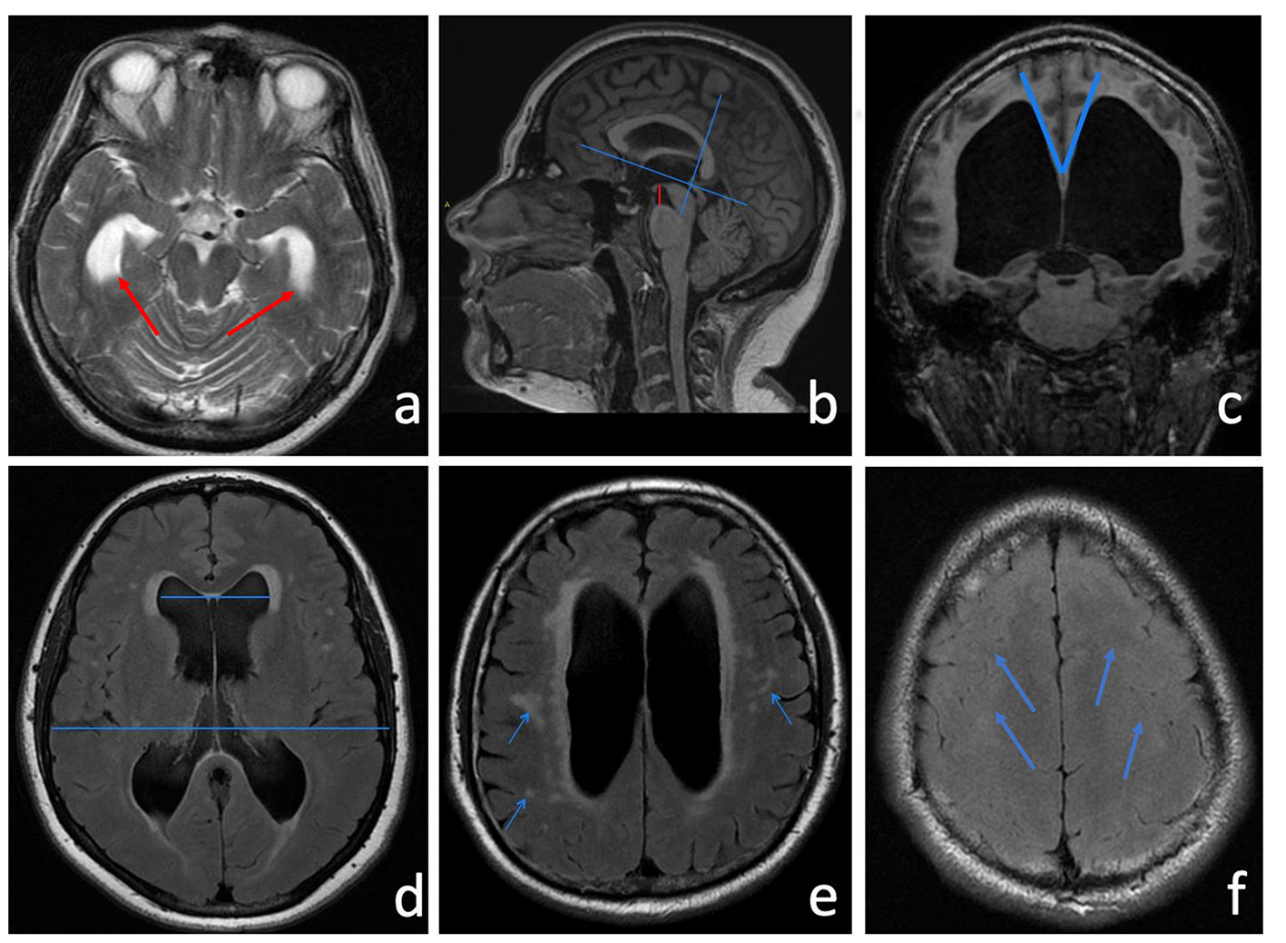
(a) Axial T2-w showing a clear enlargement of the temporal horns (red arrows). (b) Sagittal 3D MPRAGE T1-w was used to measure the height of the interpeduncular cistern, which represents the shortest distance between the floor of the third ventricle and the midbrain (a red line). After identifying the AC-PC plane, the callosal angle (c) is measured in the coronal plane through the posterior commissure perpendicular to the anterior commissure-posterior commissure (AC-PC) plane. Neuroradiological features of LIAS mimic iNPH also for CA amplitude that usually measures <90°. (d) Evan's index (EI) is a direct linear measurement of the ventricular size. It is calculated from the ratio between the maximum transverse diameter of the frontal horns and the maximum internal diameter of the skull. (e) The FLAIR sequence shows an elevated periventricular signal ascribable to interstitial edema or reactive gliosis. Hyperintense white matter lesions attributed to chronic ischemia of the small vessels are indicated by the arrows. (f) Narrowed sulci at the high cerebral convexities (blue arrows).
